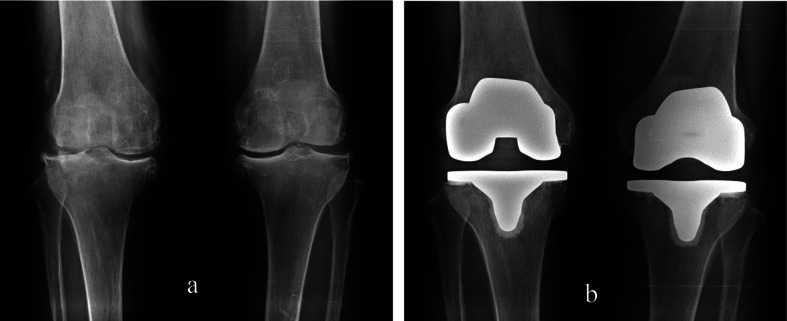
(a) Preoperative both knee radiographs reveal narrowing of articular space with subchondral sclerosis and spur formation. (b) Postoperative both knee radiographs reveal metallic prosthesis in both knee joints.
